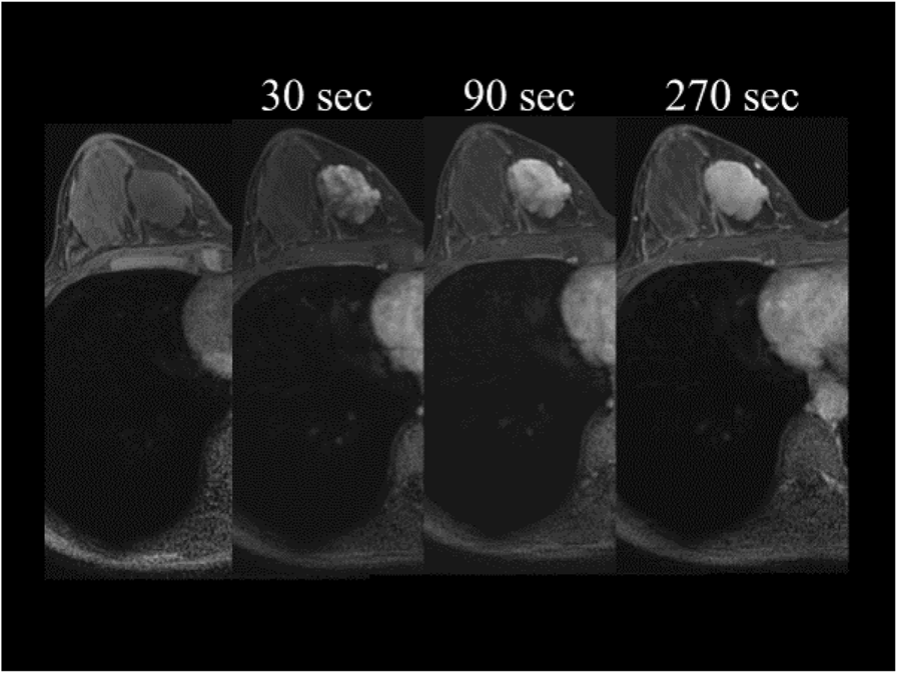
MRI showed a gradually enhanced, well-circumscribed tumor
